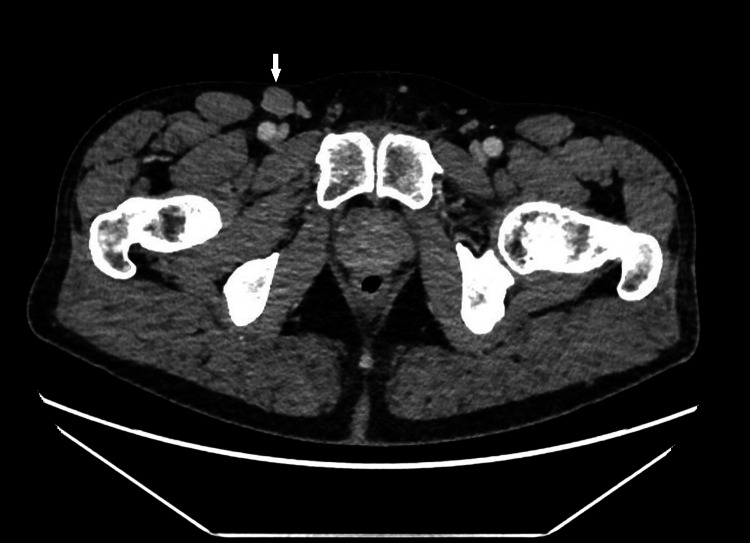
A transversal slice of CT scan of the abdomen revealing a 17 mm right inguinal lymph node (arrow).
